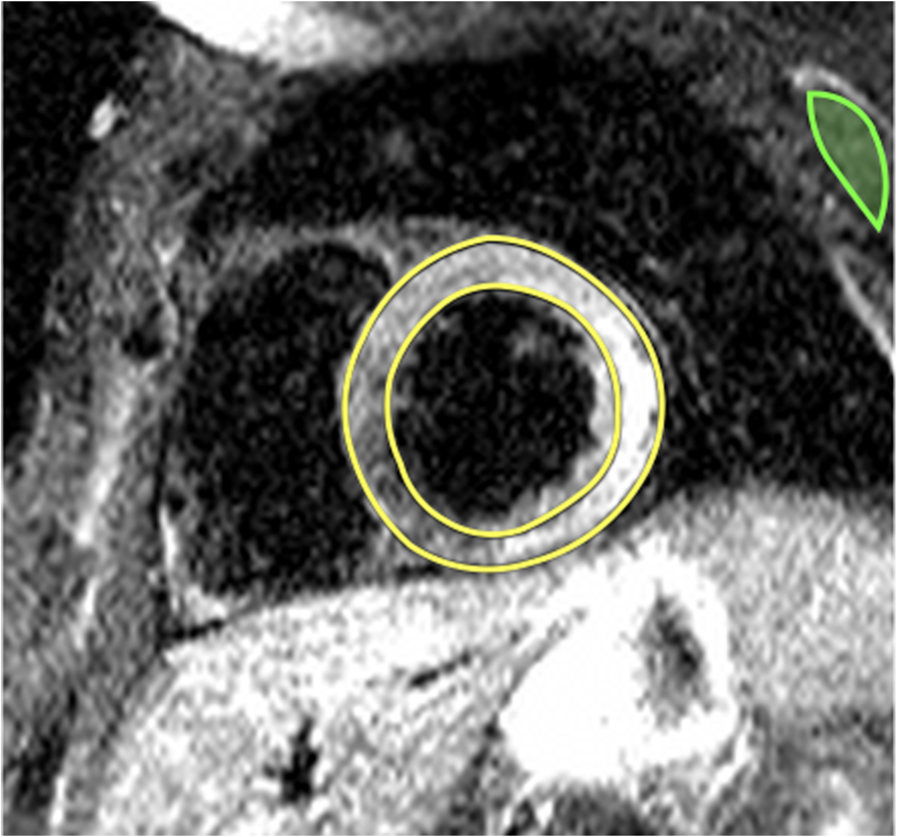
T2-weighted image (short-tau inversion recovery, STIR) in a midventricular short axis view with increased SI in the inferolateral and lateral segments in acute myocarditis
